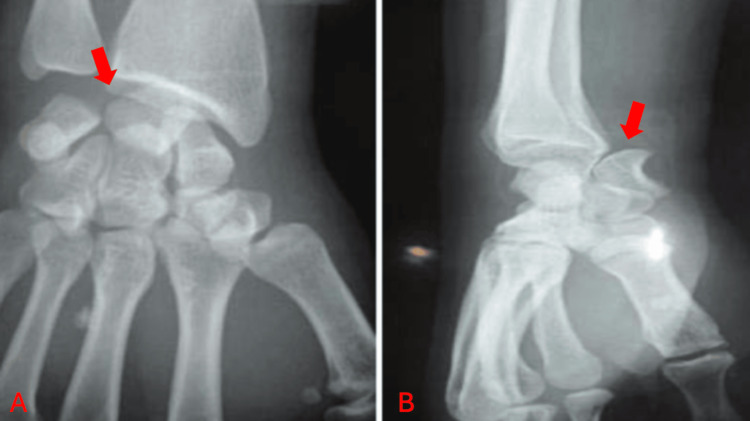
Anteroposterior and lateral wrist radiographs showing a pure perilunate dislocation classified as Herzberg stage IIb with dorsal displacement of the capitate (A) Anteroposterior view showing disruption of the normal carpal alignment with lunate dislocation (red arrow). (B) Lateral view demonstrating dorsal displacement of the capitate relative to the lunate (red arrow).
